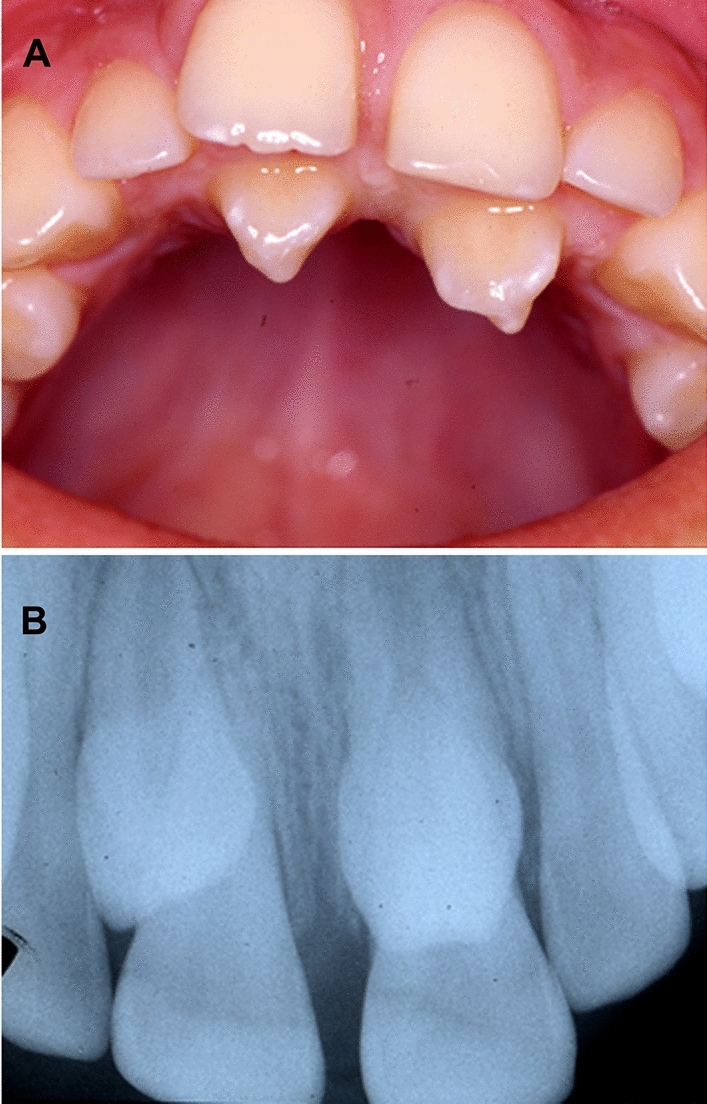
Clinical (a) and radiographic (b) features of erupted, supplemental multiple supernumerary teeth in palatal position
